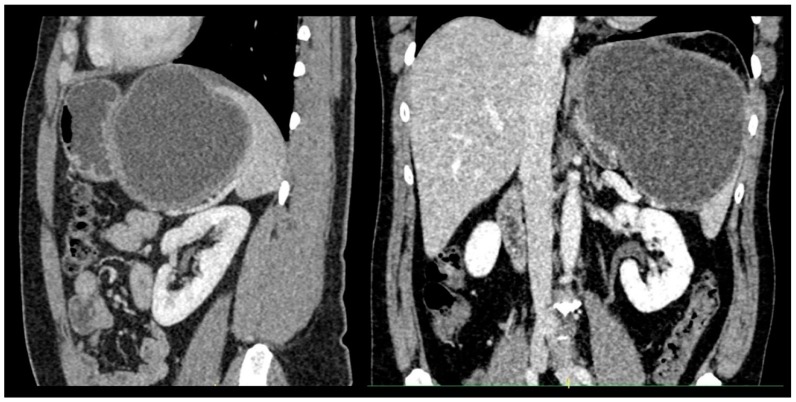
Abdominal computed tomography showing a large splenic cyst.
